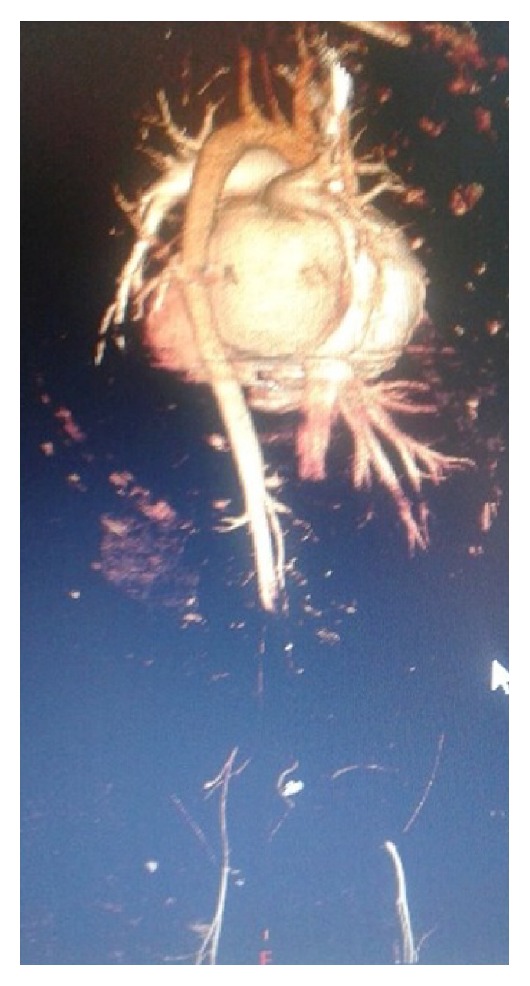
Abdominal computed tomography with contrast demonstrated a large occlusive thrombus within the infrarenal aorta, thrombus in bilateral iliac artery, and focal hypodense areas in the left external right kidney parenchyma.
